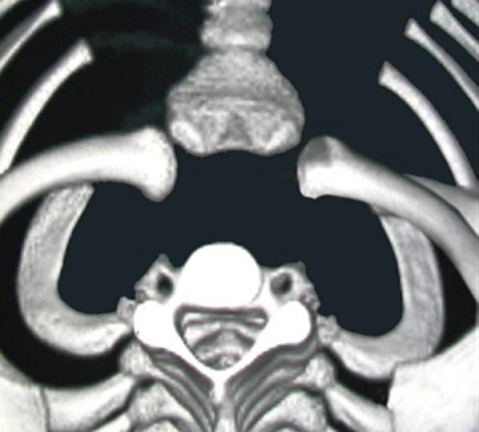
Chest CT scan showing reduction
